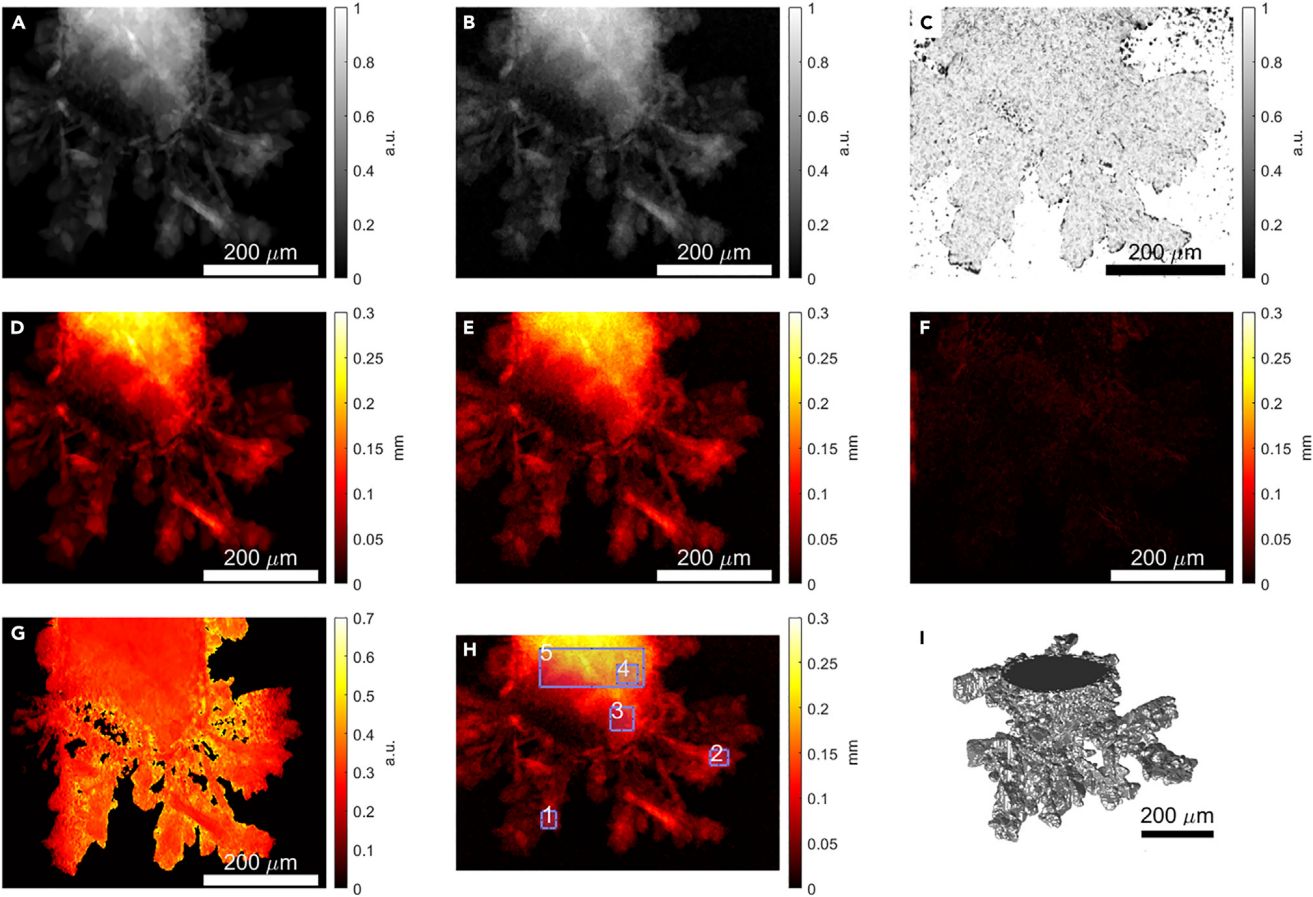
Comparison between radiographic and tomographic data Electrode image acquired as radiogram (A) and from forward projected, segmented 3D reconstruction (B) shows good overlap after PC registration as indicated by local SSI map (C)Thickness maps calculated from radiogram (D) and obtained from 3D data (E) are matching as well (F). Note that calculated proportionality factor d/I_n_ exhibits little noise at the edges (G), therefore 5 regions were selected (I) to assess local differences. 3D render (H) is presented to show that the dendrites are indeed three-dimensional.
